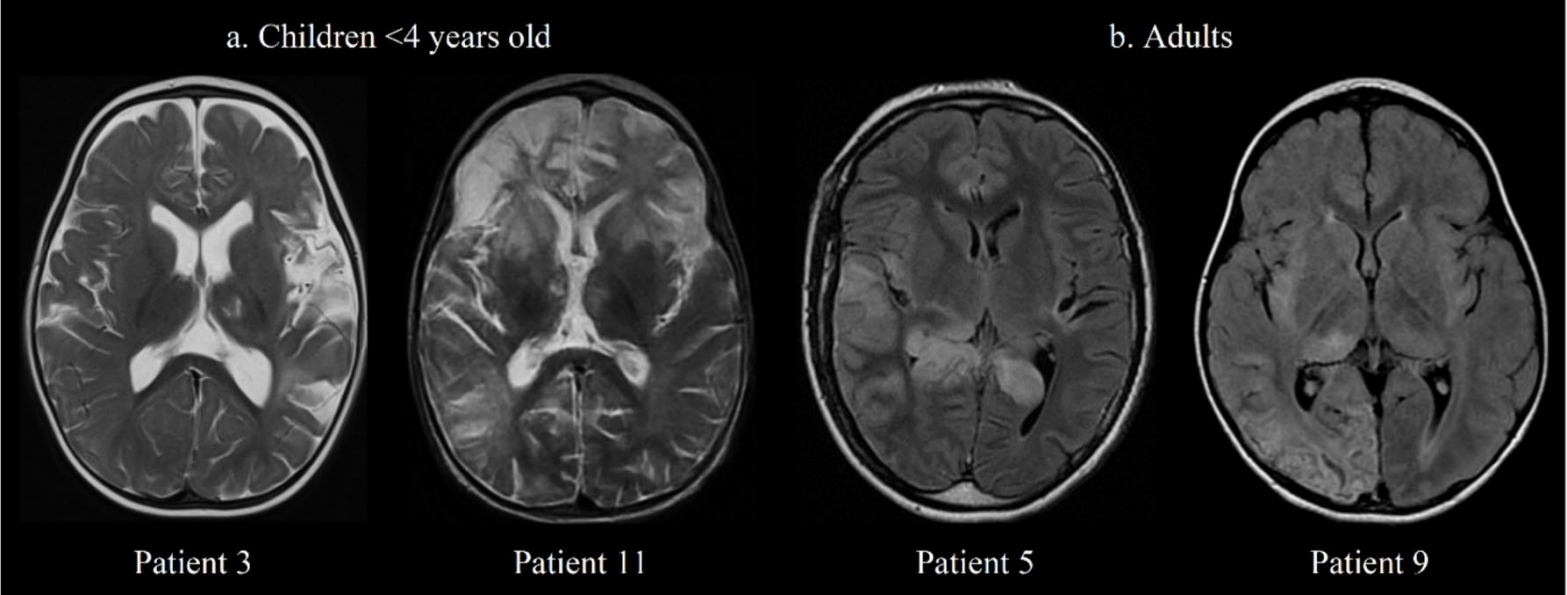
Topography of thalamic lesions in patients with HSE-NMDARE. The characteristics of thalamic lesions are shown for 4 patients at NMDARE diagnosis, according to their age. On the left (a), T2 sequence shows median/anterior thalamic lesions in patients 3 and 11, who were children < 4 years old. On the right (b), FLAIR sequence shows hyperintensity in the pulvinar region in adult patients 5 and 9. Abbreviations: *FLAIR* T2-fluid-attenuated inversion recovery, *NMDARE* anti-*N*-methyl-d-aspartate receptor encephalitis
